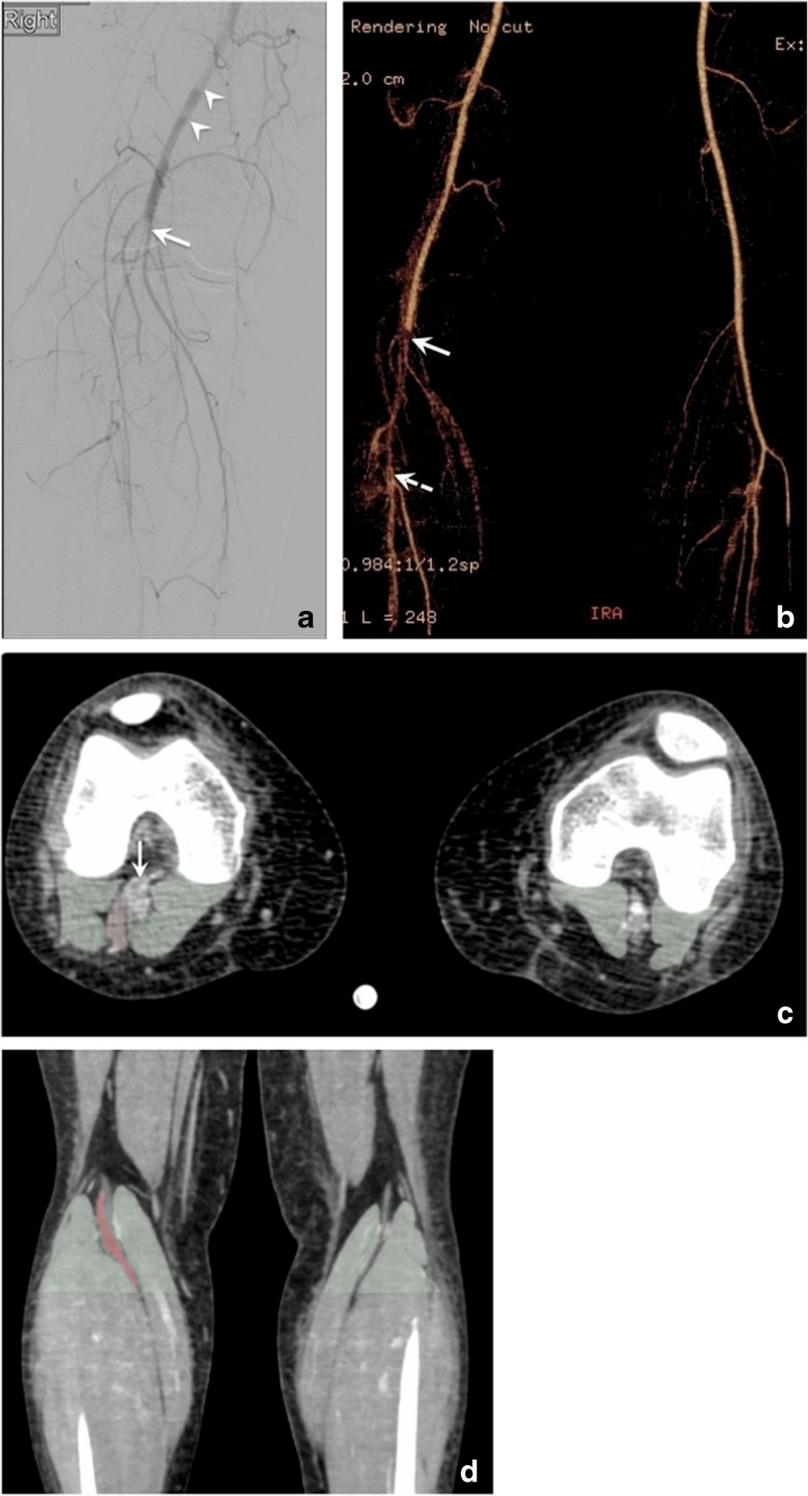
Popliteal artery entrapment syndrome (PAES) in a 38-year-old woman with a 4-week history of right foot pain, admitted to the hospital for gangrenous fourth toe. (a) Digital subtraction angiogram shows total occlusion of the right popliteal artery (*arrow*), with filling defects of the distal superficial femoral artery (*arrowheads*) consistent with thrombus, and multiple collaterals around the knee. PAES was suspected and patient underwent CT angiogram. (b) Maximum-intensity projection reconstruction from lower extremity CT angiogram demonstrated total occlusion of the right popliteal artery (*arrow*) extending into the proximal anterior tibial artery, with distal reconstitution (*dashed arrow*). (c) Axial CT angiography image shows occlusion of the right popliteal artery (*arrow*), and an extra slip of muscle lateral to it (*red transparent overlap*). No comparable structure is seen on the left side, which suggests right PAES. *Green transparent overlapping areas* of the normal gastrocnemius heads bilaterally are added to display the asymmetrical appearance between right and left knees. (d) Coronal reconstruction of CT angiography showing the gastrocnemius heads (*green transparent overlap*) with an extra slip of muscle (*red transparent overlap*) causing asymmetric appearance, and possibly originating from the lateral head
